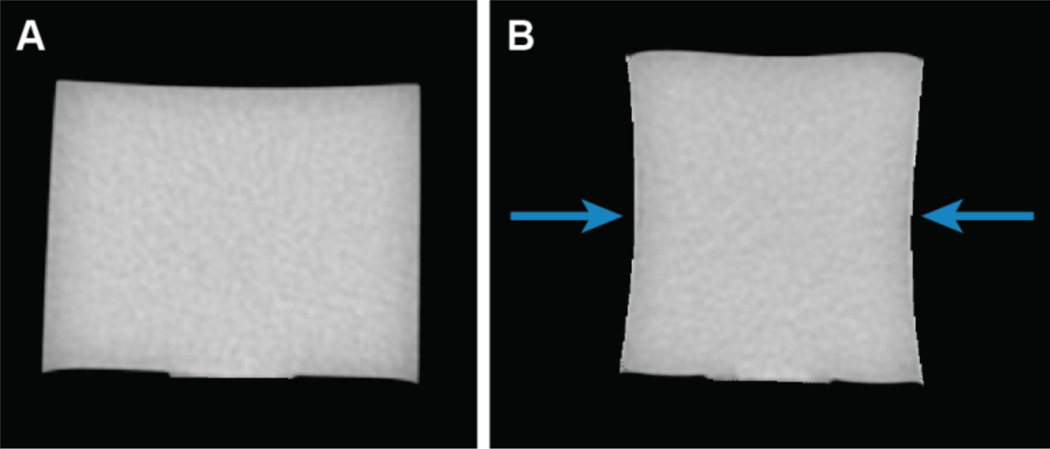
(A) CT of 6 cm diameter dosimeter without compression. (B) CT of the same dosimeter with compression up to 1.6 cm (27%). Arrows show direction of compression.
